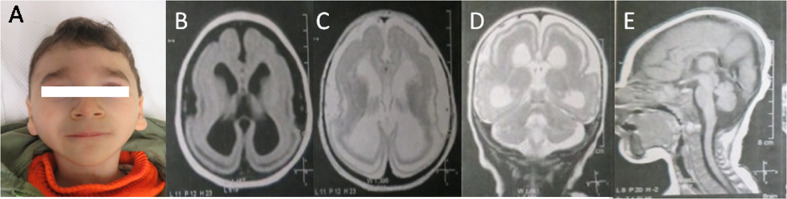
Our Patient at the age of 24 months (A) Note the thick eyebrows, upturned nostrils and microretrognathia. The brain MRI (B–E) showed lissencephaly with cell sparse zone, mild cerebellar hypoplasia, dysplastic hippocampus, hypoplastic corpus callosum and mild ventricular dilatation
